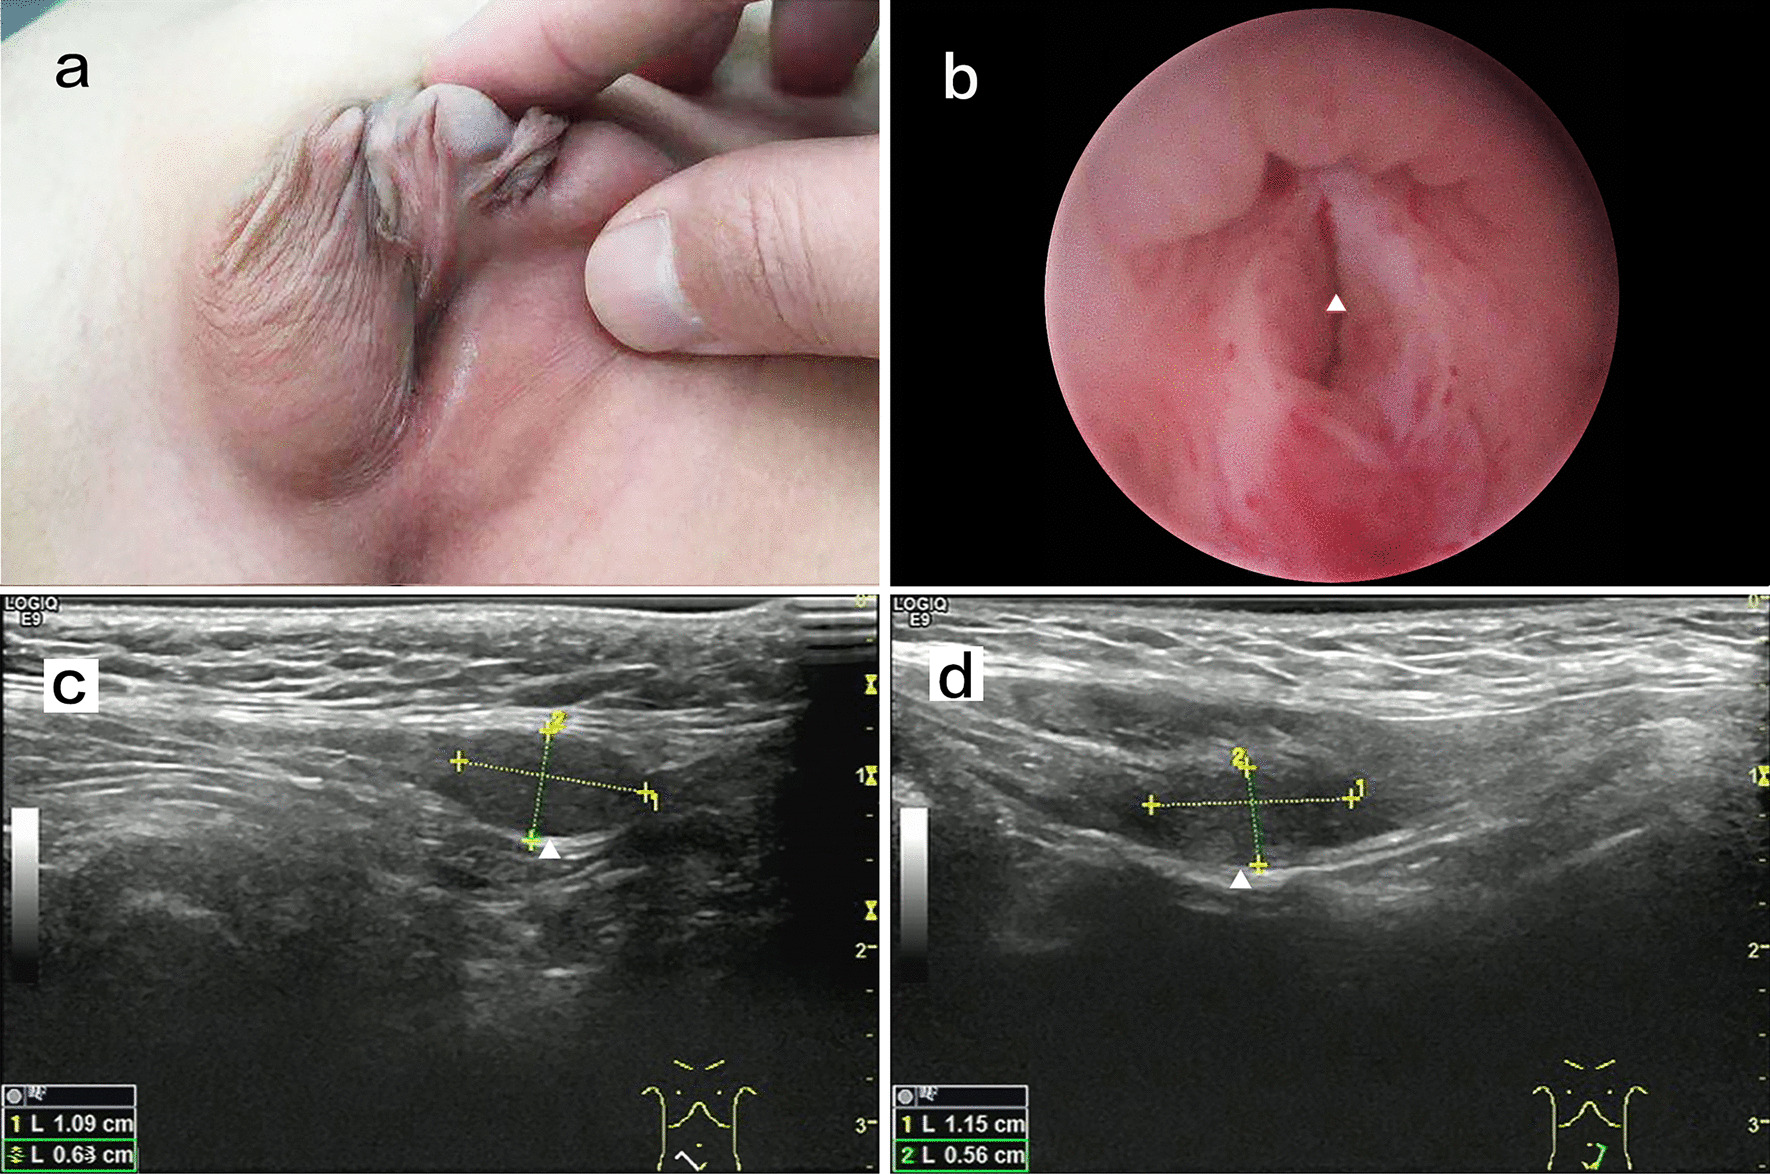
a Physical examination revealed scrotal hypospadias and bilateral cryptorchidism. b Pre─operation cystoscopy showing an orifice in the prostatic urethra; the white arrow indicates the prostatic urethra orifice. c Ultrasonography showing that the right testicle is in the inguinal canal; the morphology was normal. d Ultrasonography showing that the left testicle is in the inguinal canal; the morphology was normal
